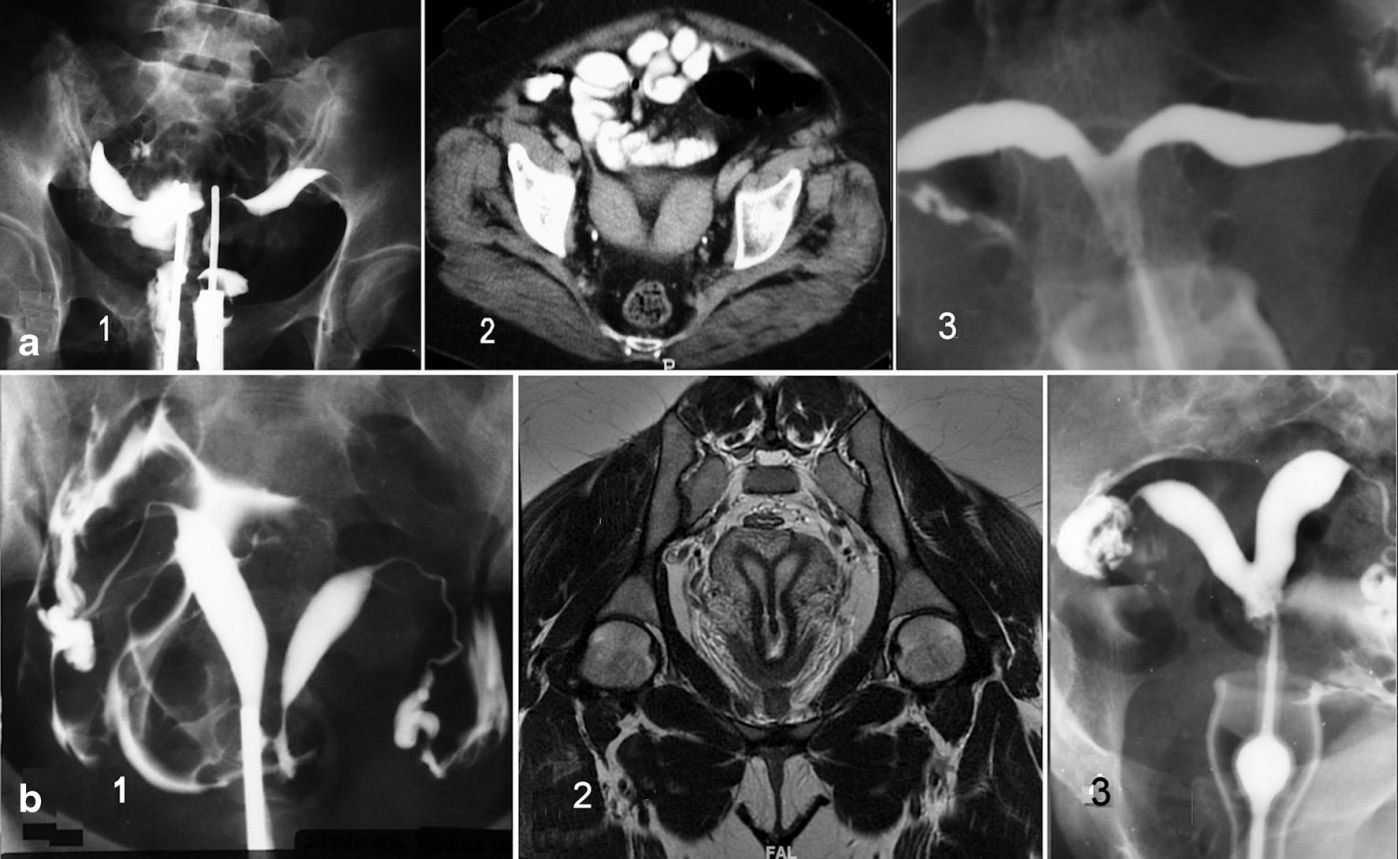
Didelphys, bicornuate and septate uteri. a 1. HSG image of a patient with didelphys uterus obtained using a double simultaneous cannula. 2. CT of other patient showing a bicornuate uterus. 3. HSG image of a bicornis-unicollis uterus. b
